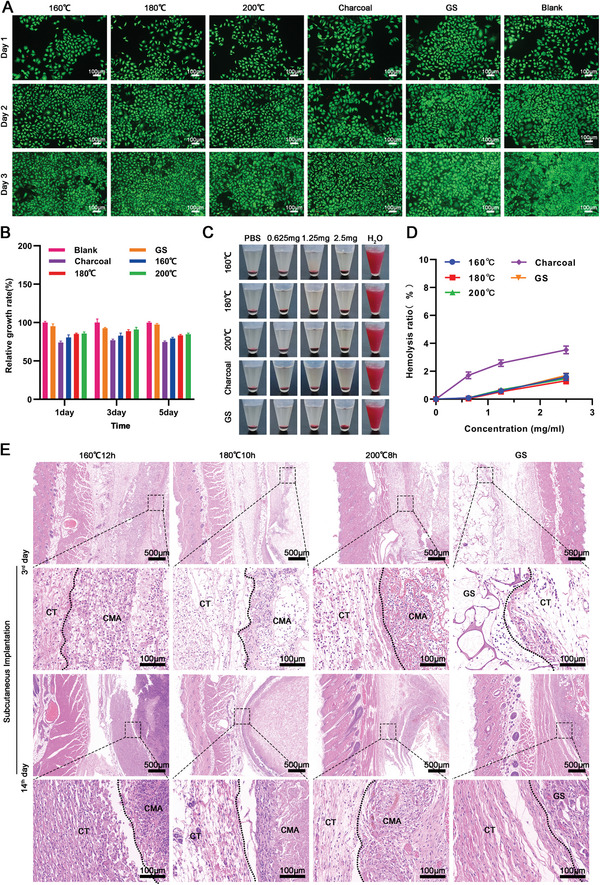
Biocompatibility evaluation of the carbonized mushroom aerogels in vitro. A,B) The proliferation and cell viability of L929 cells that co‐cultured with extract medium of different carbonized mushroom aerogels for 1, 2, and 3 days, respectively. C) The photographs of hemolysis assay of the charcoal, gelatin sponge, and carbonized mushroom aerogels with different concentrations, ranging from 2.5 to 1.25, and 0.625 mg mL^−1^. D) The quantification of the hemolysis assay. CT: connective tissues. CMA: carbonized mushroom aerogels.
